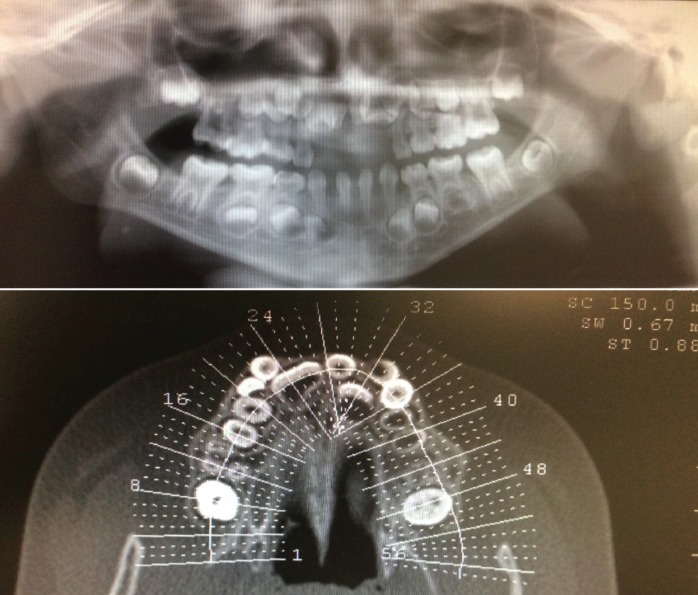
Orthopantomography and computer tomography. Both were performed with a diagnostic purpose prior to the surgery of mesiodens extraction.
